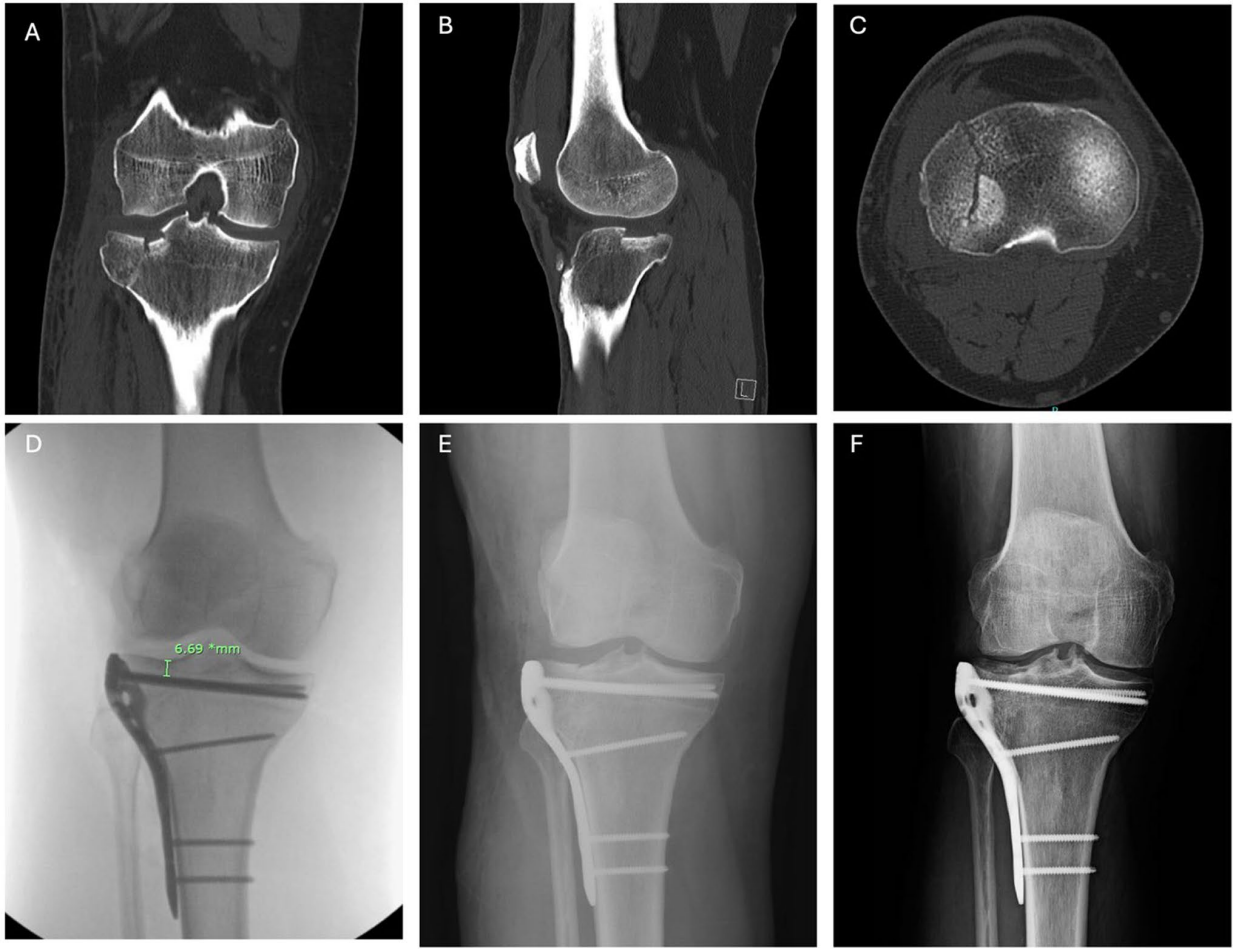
A 60-year-old male sustained this fracture after a ground-level fall and received a standard 3.5-mm anterolateral plate. Preoperative computed tomography demonstrates a right tibial plateau lateral split-depression fracture in the a coronal, b sagittal, and c axial planes. d The SJD, as measured on intraoperative fluoroscopy, is 6.69 mm. e Immediately postoperatively. f Final follow-up at 55 weeks postoperatively shows maintenance of articular congruity and healing
